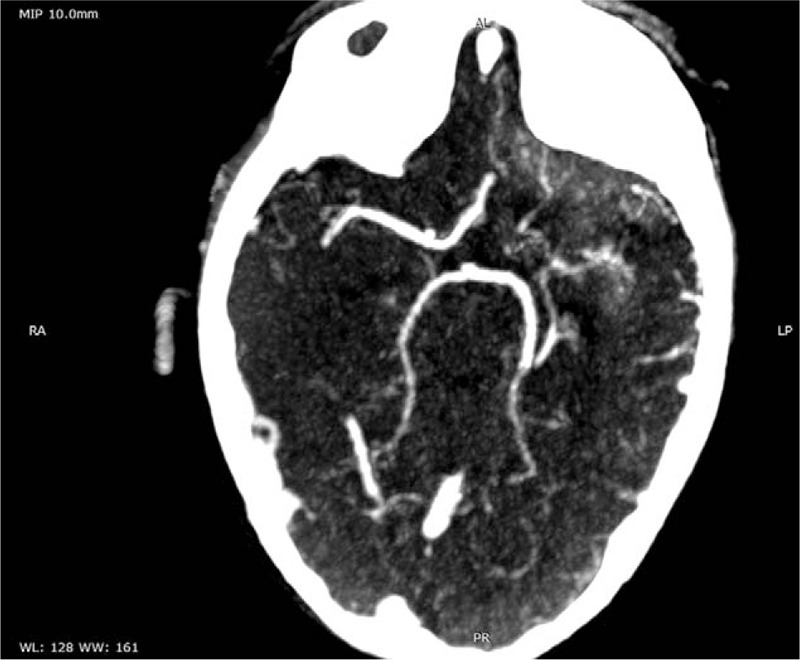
Angio CT, axial section.
